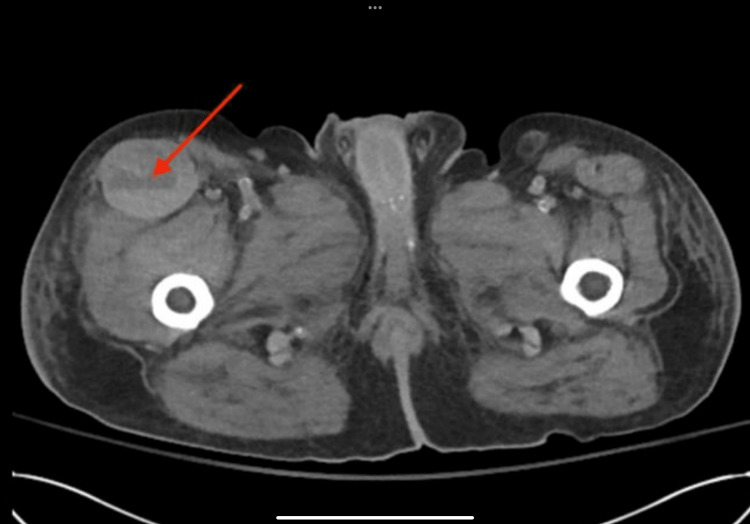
A pelvic CT scan without contrast detected a sizable fluid collection in the right groin to medial aspect of the thigh, suggesting hemorrhagic myositis in a patient recently diagnosed with dermatomyositis.
